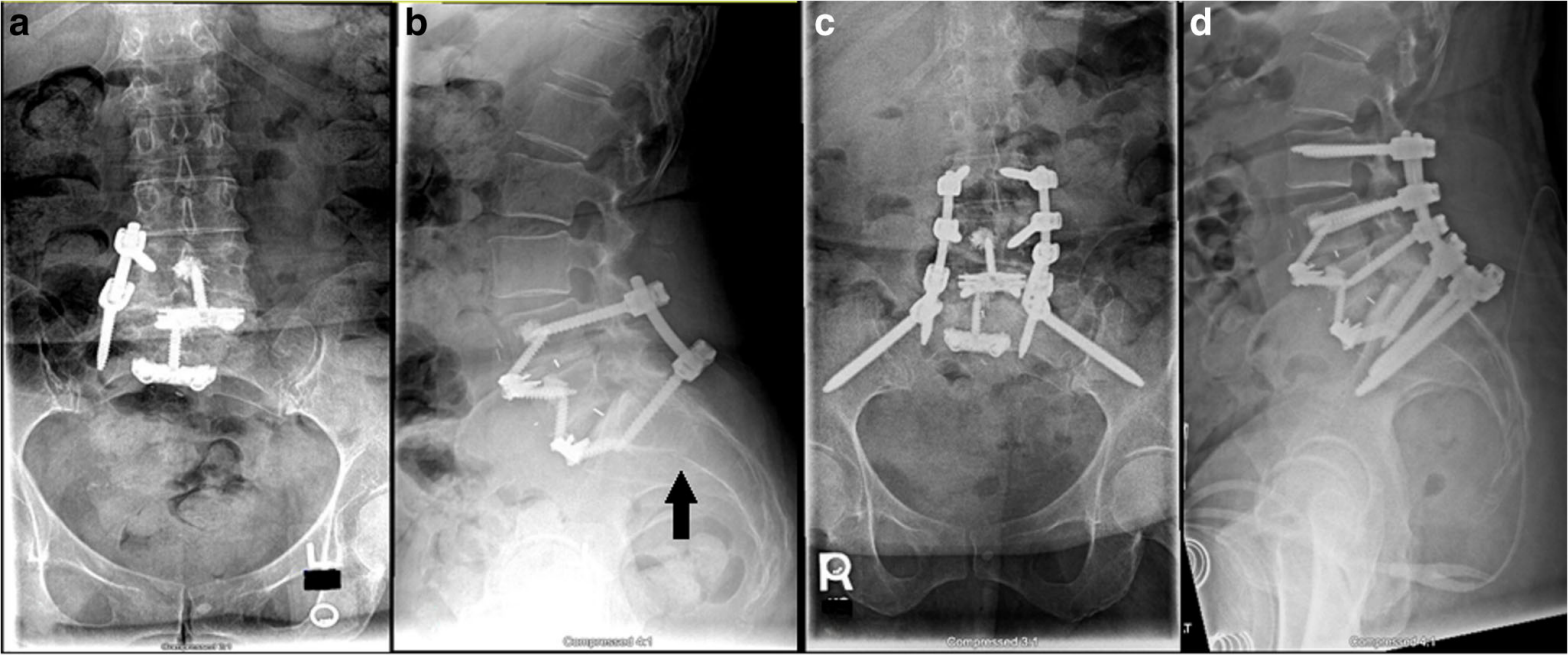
(a) Anteroposterior (AP) and (b) lateral plain radiographs showing sacral fracture (black arrow). (c) AP and (d) lateral plain radiographs taken after revision surgery.
